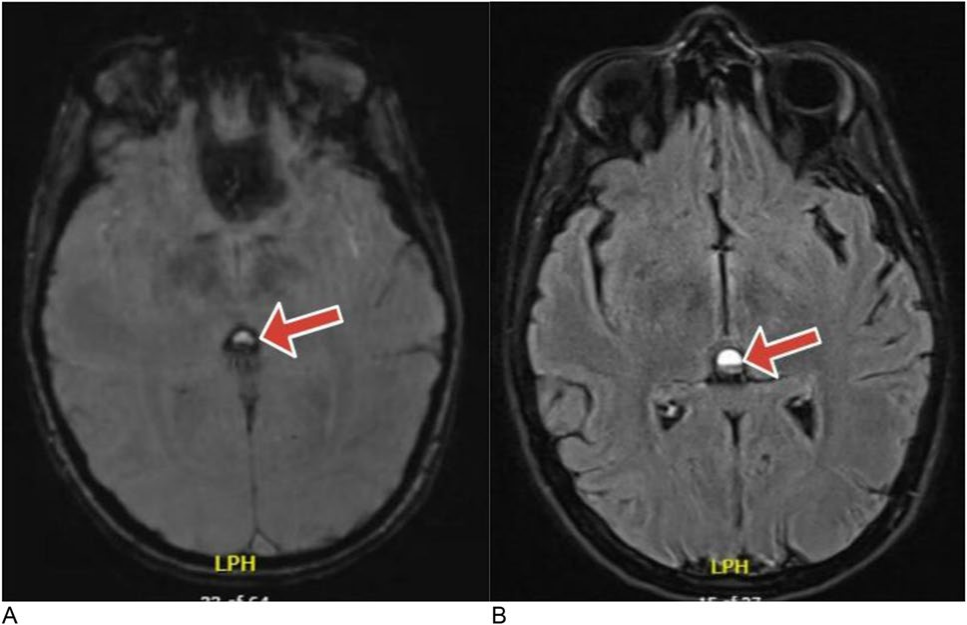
(A) Susceptibility weighted imaging (SWI) MRI without contrast showing an evidence of layered hemorrhagic components in the pineal cyst. (B) Fluid-attenuated inversion recovery (FLAIR) MRI showing mixed intensity blood products inside the pineal cyst
